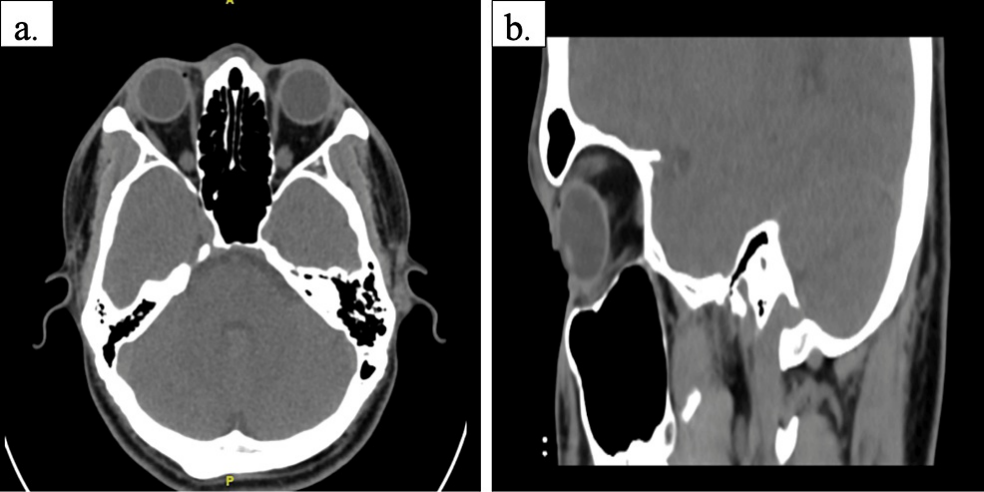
Plain computed tomography of the orbit showed no detectable intraocular radiopaque foreign body in both axial (a) and sagittal (b) views.
